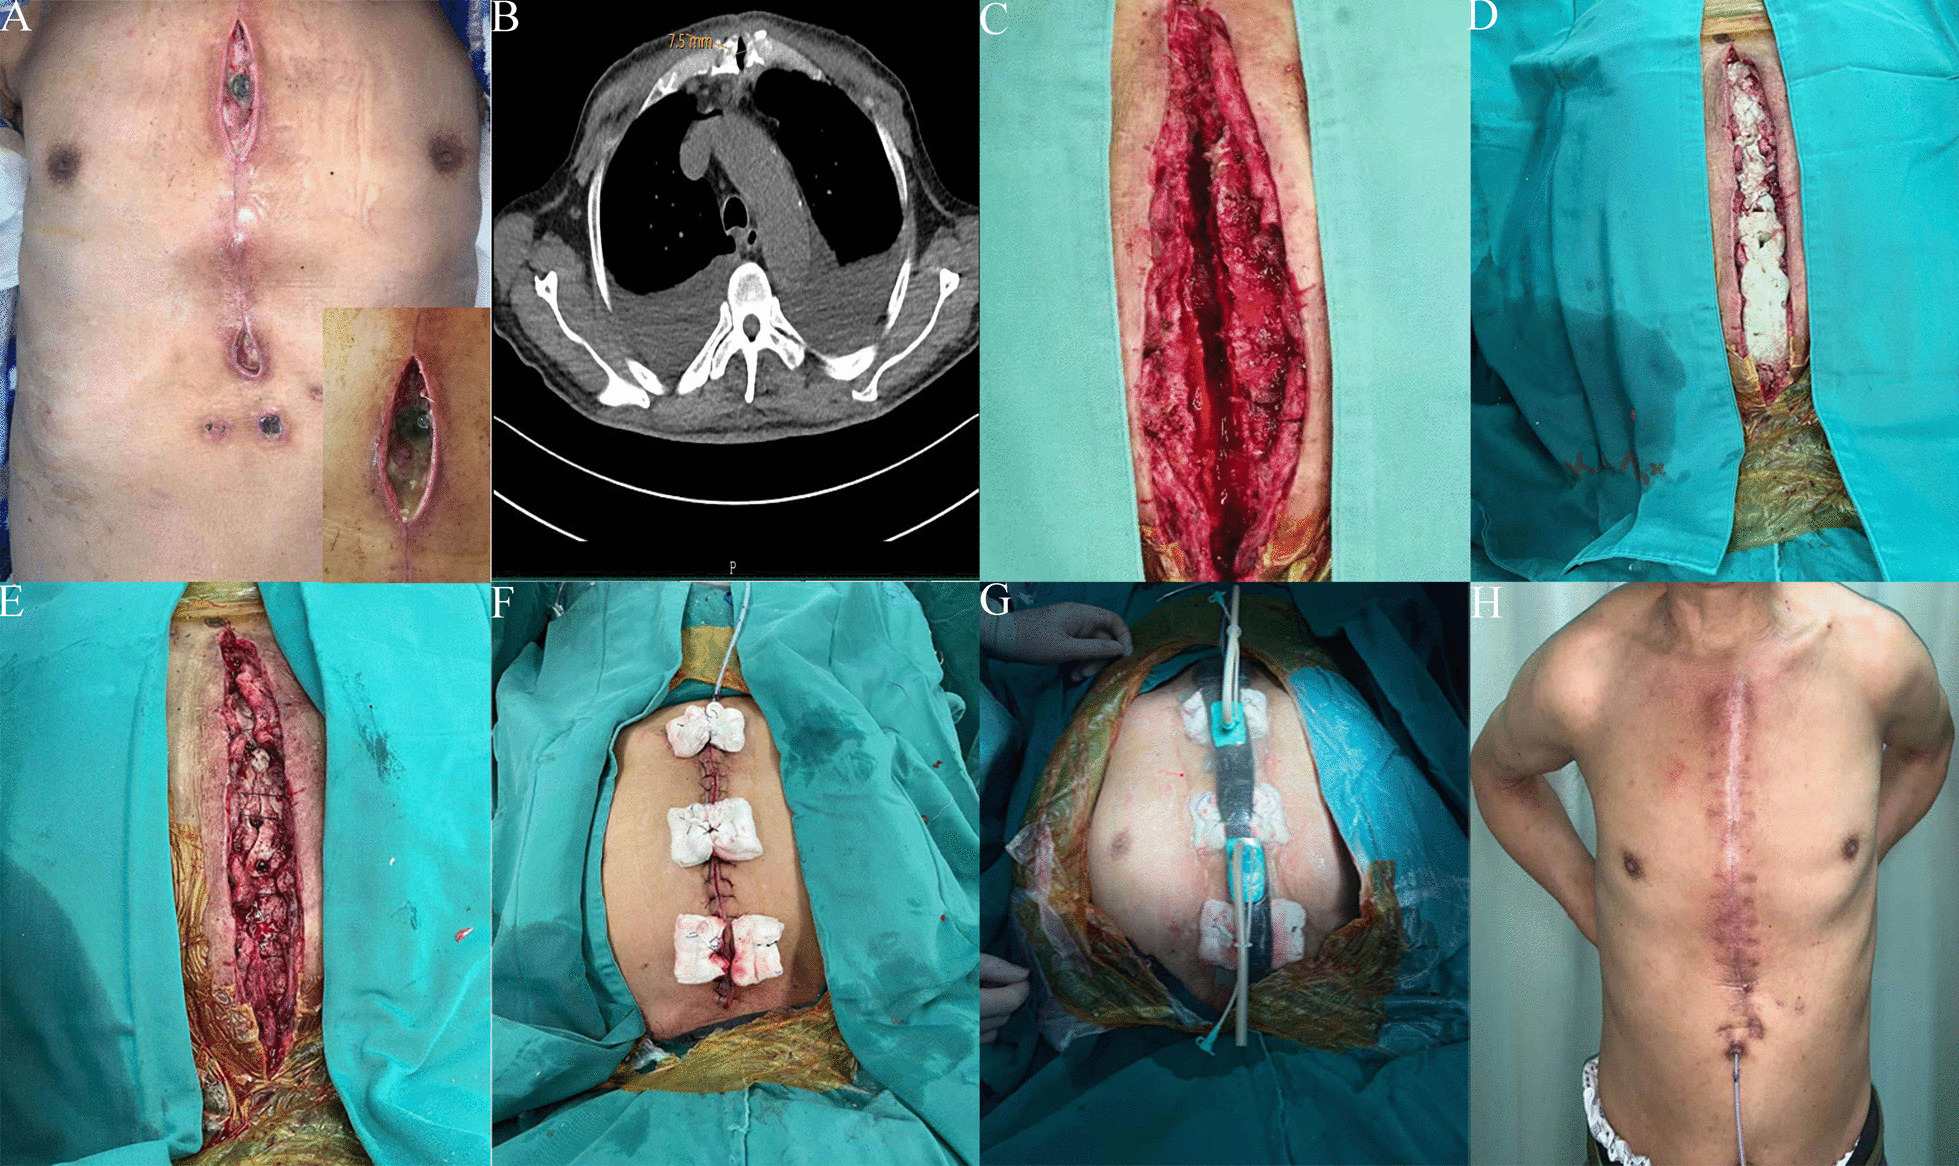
I-stage surgical procedure of the DSWI. a Post-sternotomy wound infection; b Chest CT revealed sternal dehiscence and non-unionc; c The wound after debridement; d ALBC was inseted on the sternal defect; e Mobilization of pectoralis major muscle flap and intermittent sutures; f Deep sternal wound infection-associated defect after reconstruction and skin suture; g VSD coverage; h 4th postoperative week
